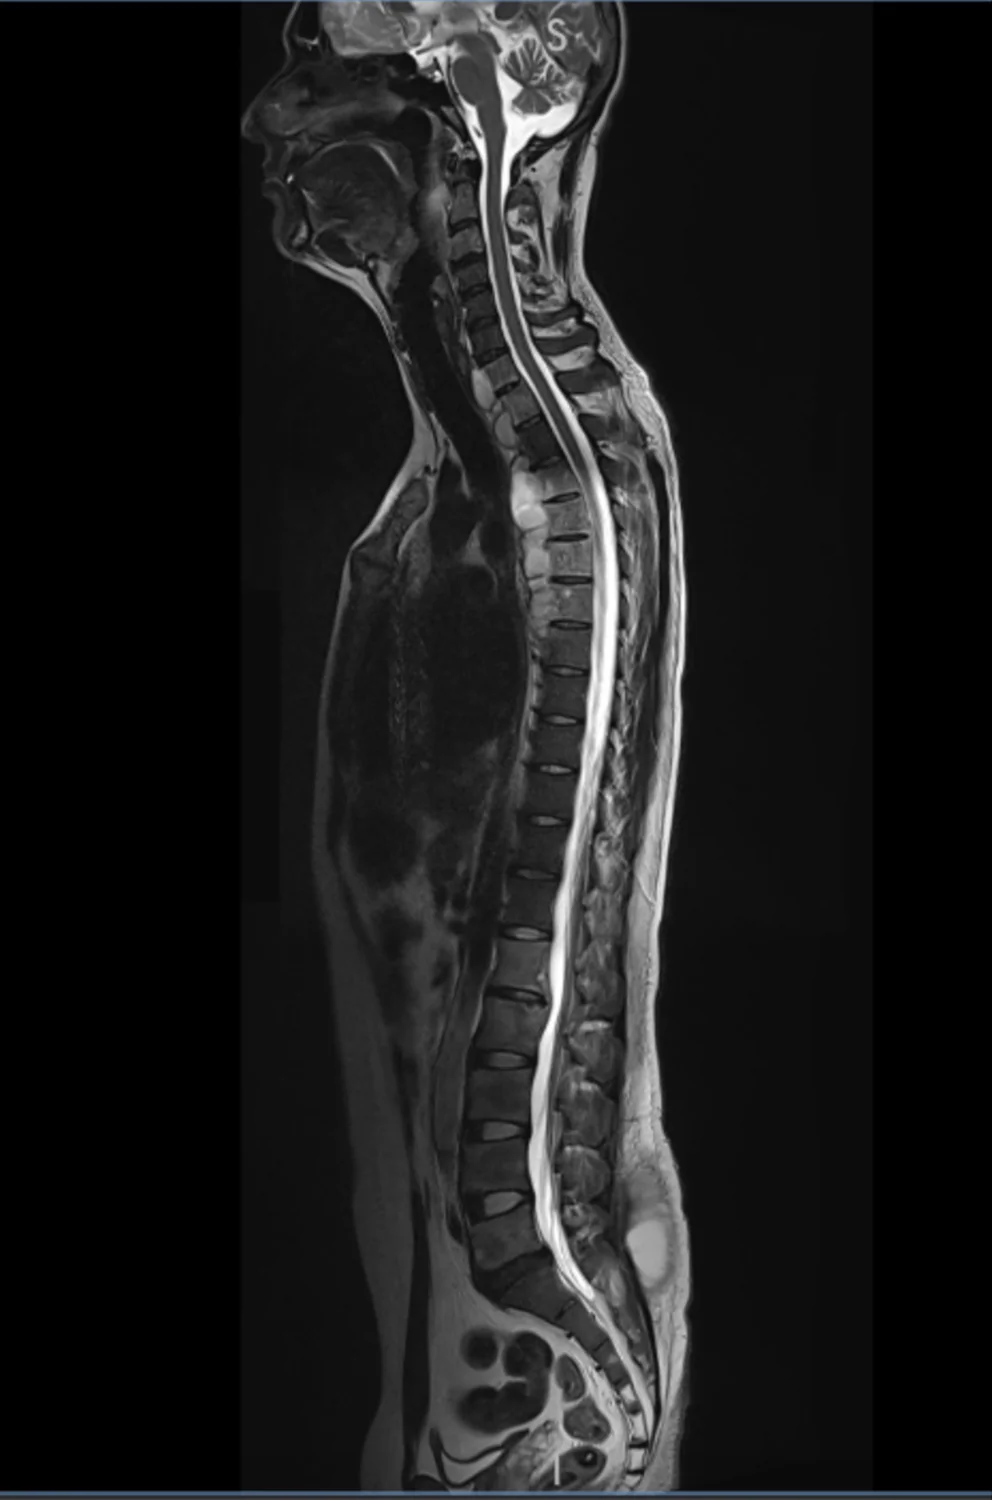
Sagittal T2‐weighted MRI of the whole spine showing hyperintense paraspinal collection in the lumbosacral region consistent with cold abscess, with associated vertebral involvement suggestive of tuberculous spondylodiscitis.
